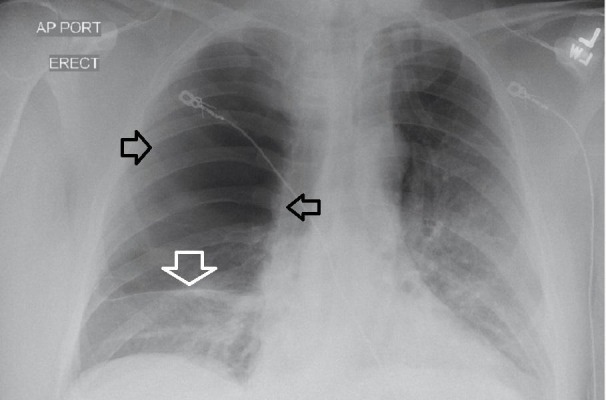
Chest radiograph on admission. Lung parenchyma is not apparent in the right hemithorax consistent with pneumothorax (black arrows). However, this is bullous disease occupying most of the right hemithorax. The remaining right lung tissue at the bottom of the lung field has an opacity (white arrow).
